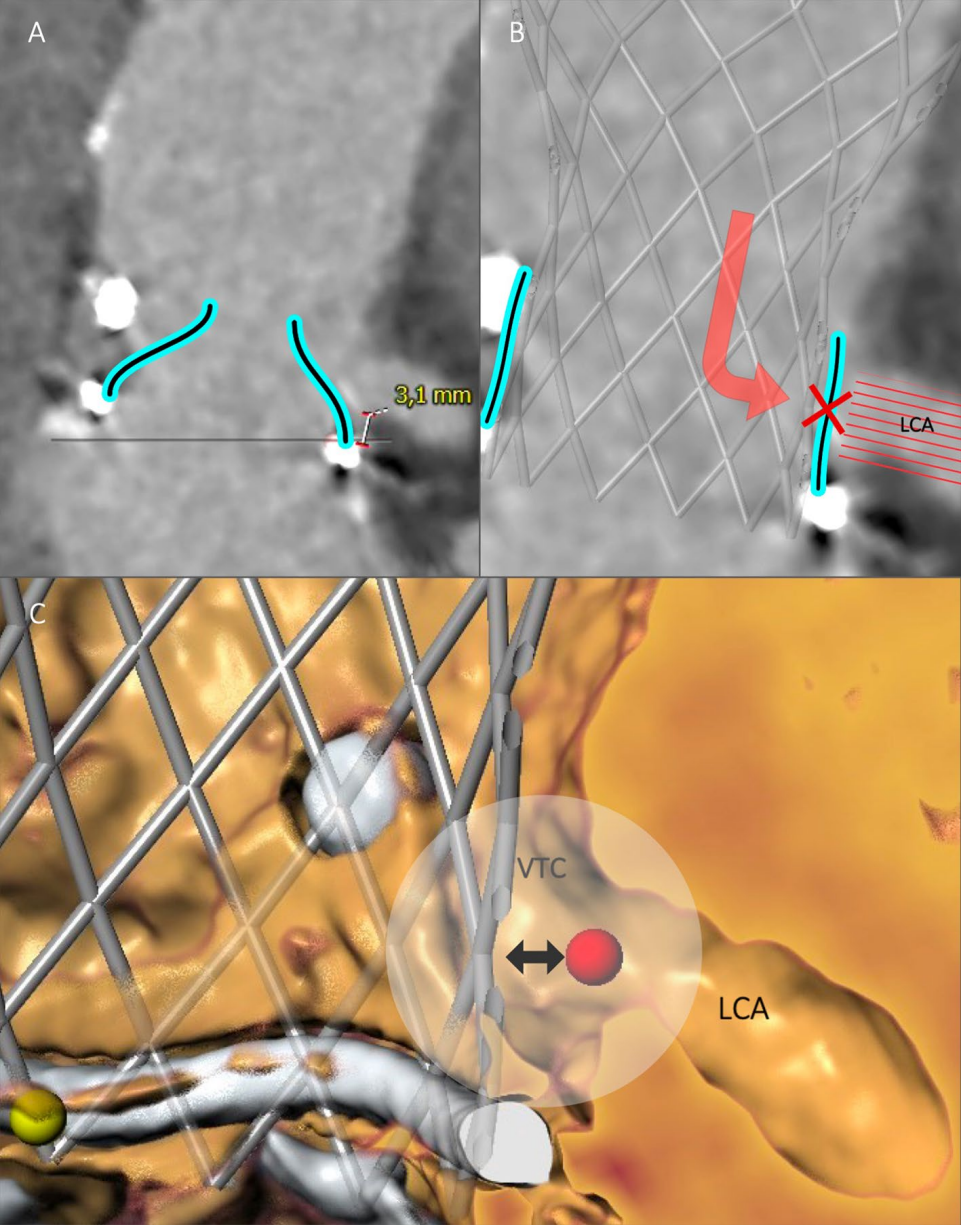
Deficient Sinus. 3mensio Structural Heart V9.1, Pie Medical Imaging, Maastricht, Netherlands. A Deficient Sinus (pre-procedural CT). Deficient Sinus is defined by low coronary height and narrow sinus of Valsalva. Measurement (3.1 mm) indicates low left coronary artery height. Surgical valve leaflets have been drawn retrospectively. B Coronary obstruction in a patient with Deficient Sinus [pre-procedural CT with a virtually implanted THV (Evolut R, *Medtronic*)]. Following THV implantation, the surgical valve leaflet directly obstructs the complete sinus of Valsalva, including the LCA, inhibiting coronary blood flow. C Valve-to-coronary (VTC) distance (3D CT reconstruction). *CT* computed tomography, *LCA* left coronary artery, *THV* transcatheter heart valve, *VTC* valve-to-coronary distance
